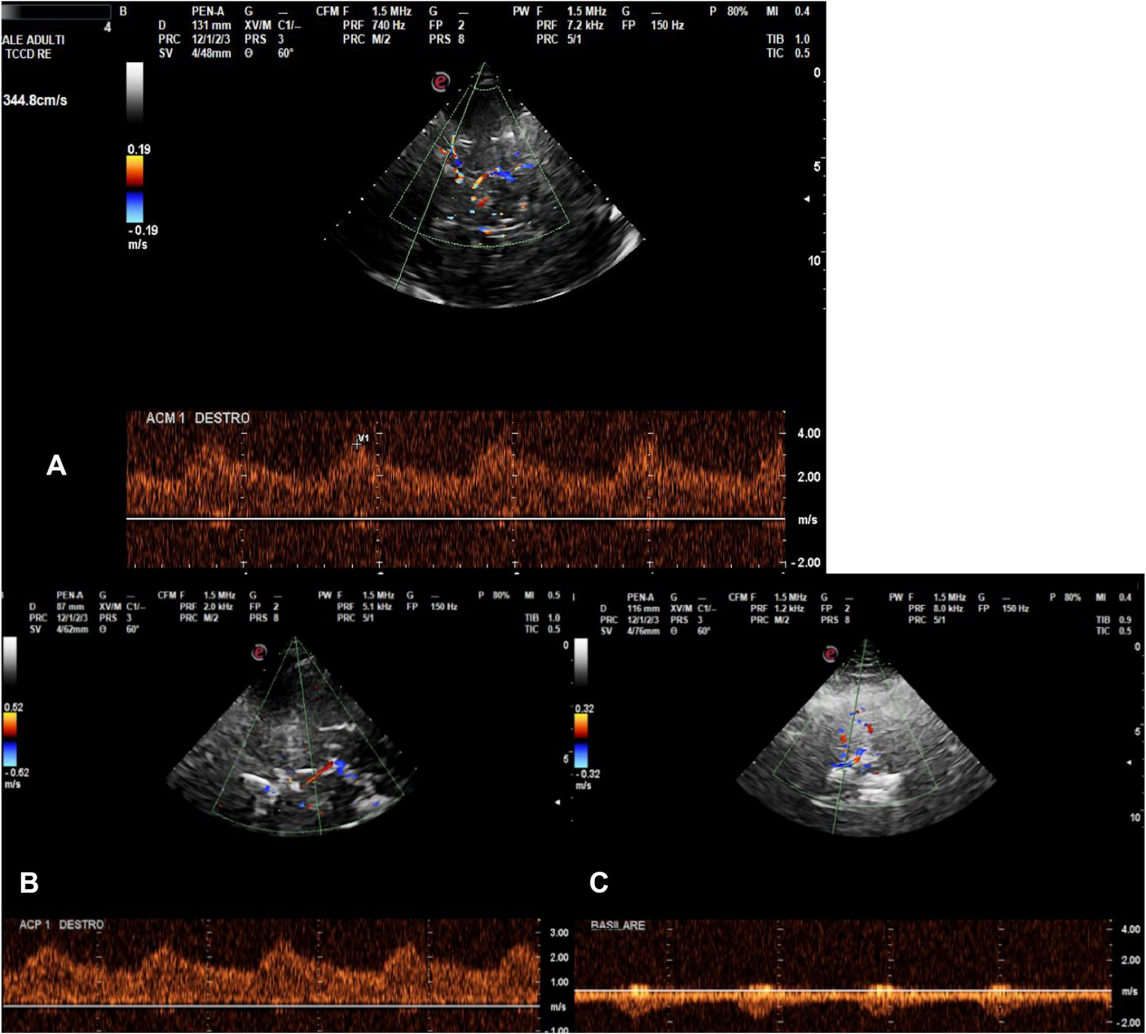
Transcranial color-coded Doppler (TCCD) images showing focal acceleration, diffuse aliasing, and flow turbulence in the principal intracranial vessels. A Right distal middle cerebral artery (M1) with peak systolic velocity (PSV) of ≈ 380 cm/s, B right posterior cerebral artery (P1) with PSV ≈ 300 cm/s, and C basilar artery with PSV ≈ 180 cm/s and flow turbulence
